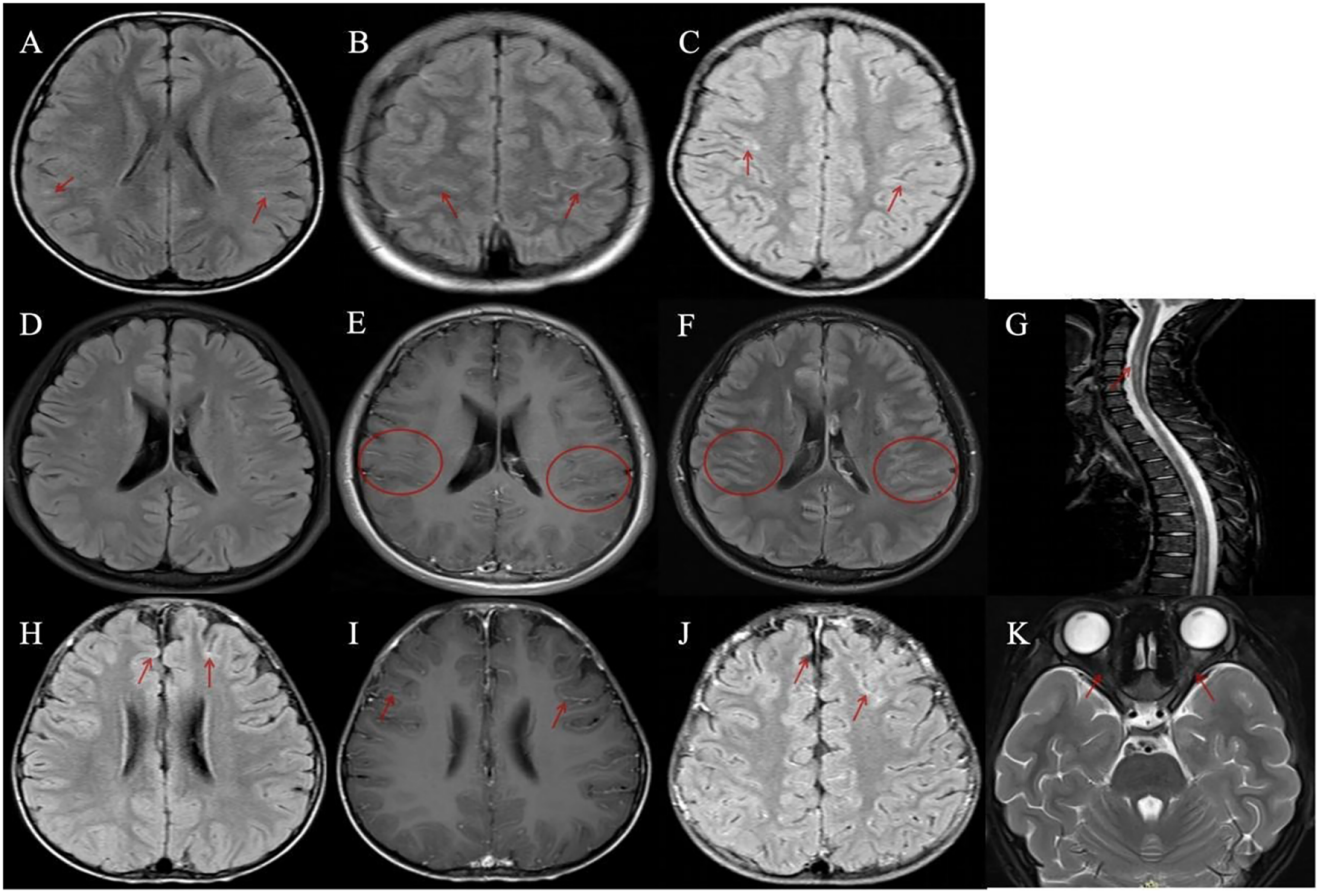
Magnetic resonance imaging (MRI) findings in patients with meningeal abnormalities and contrast enhancement. (A–C, H) Four patients demonstrated linear hyperintense signals along the cerebral sulci on fluid-attenuated inversion recovery (FLAIR) sequences. (D–K) Two patients with disease relapse exhibited leptomeningeal enhancement. (D–G) A patient with recurrent myelitis: (D) FLAIR imaging showed no abnormal lesions; (E, F) Bilateral multiple leptomeningeal enhancements were observed on gadolinium-enhanced T1-weighted axial and FLAIR images; (G) Spinal MRI revealed multiple abnormal signals involving the cervical and thoracic spinal segments. (H–K) A patient with recurrent optic neuritis: (H) A representative image showed mild linear hyperintense signals along the cerebral sulci on FLAIR sequence; (I, J) Bilateral leptomeningeal enhancements were observed on gadolinium-enhanced T1-weighted axial and FLAIR images; (K) Orbital MRI demonstrated bilateral optic nerve swelling with abnormal signals.
